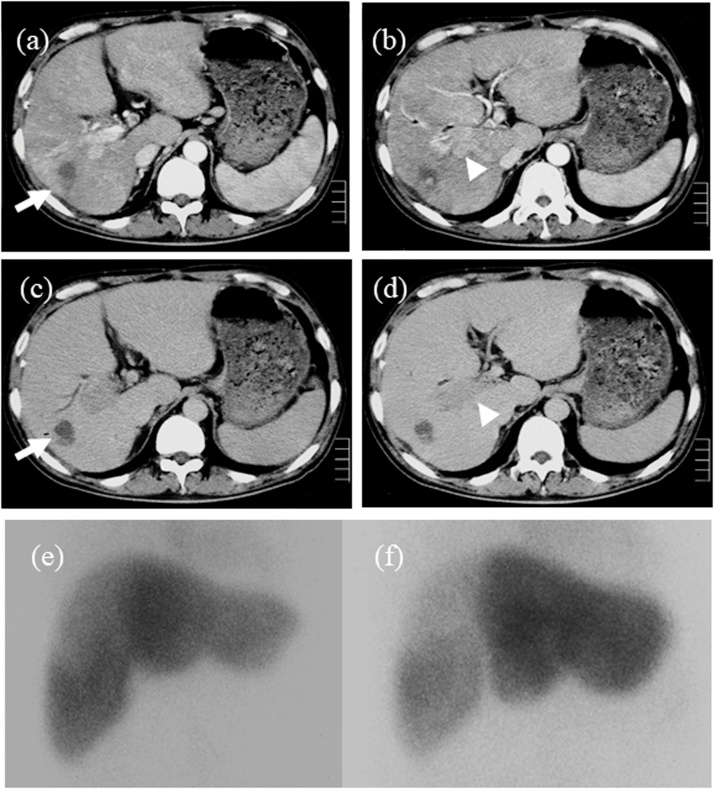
Computed tomography before the second operation showed the tumor in segment 6 decreased in size (arrow a, b: arterial phase, c, d: portal phase). However, a tumor thrombus had developed in the right main portal vein (arrow head b, d). ^99m^Tc-GSA scintigraphy revealed a reduced accumulation of GSA in the right liver (e, f).
